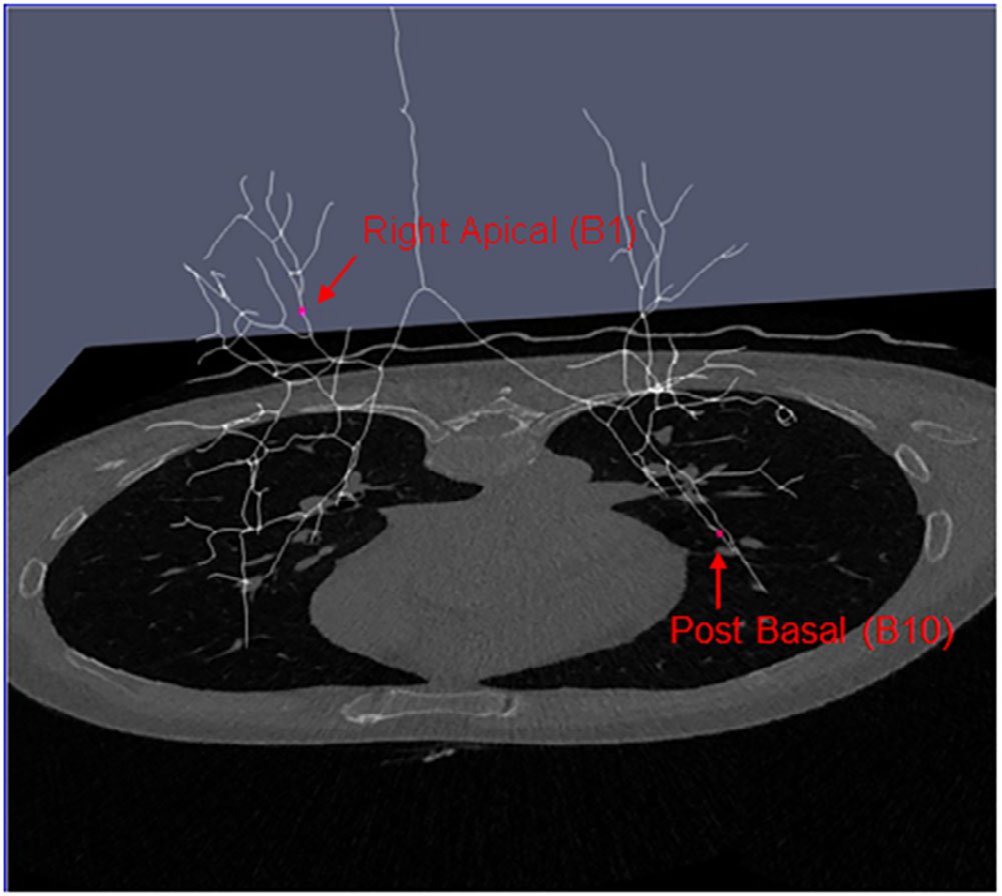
Segmental bronchi labeling. The extracted airway tree is loaded along with the corresponding CT image. Each time a segmental bronchus is identified, the annotator clicks on one point at the centerline and assigns a unique label to represent the specific segmental bronchus. CT, computed tomography.
